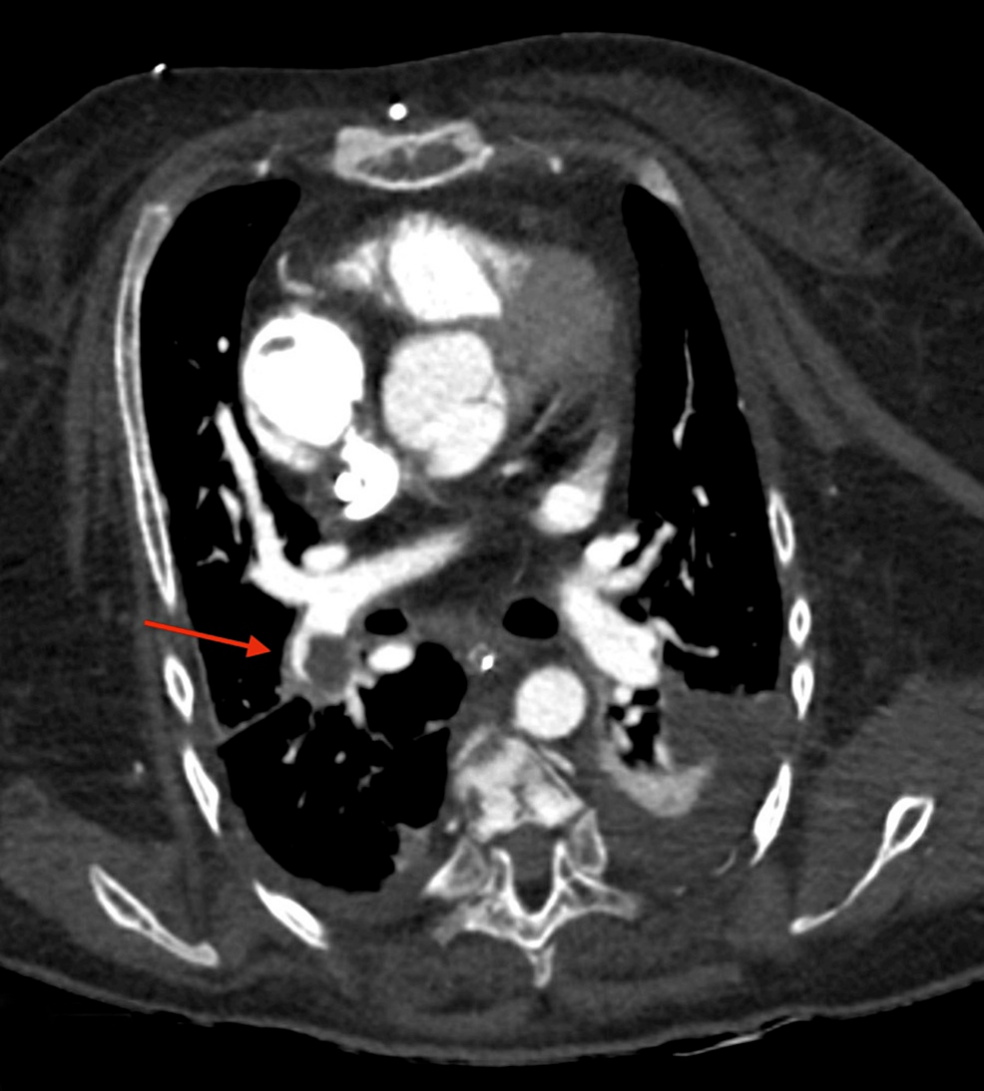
CT scan of the chest (axial view) Chest computer tomography shows the right segmental pulmonary embolism (red arrow).
